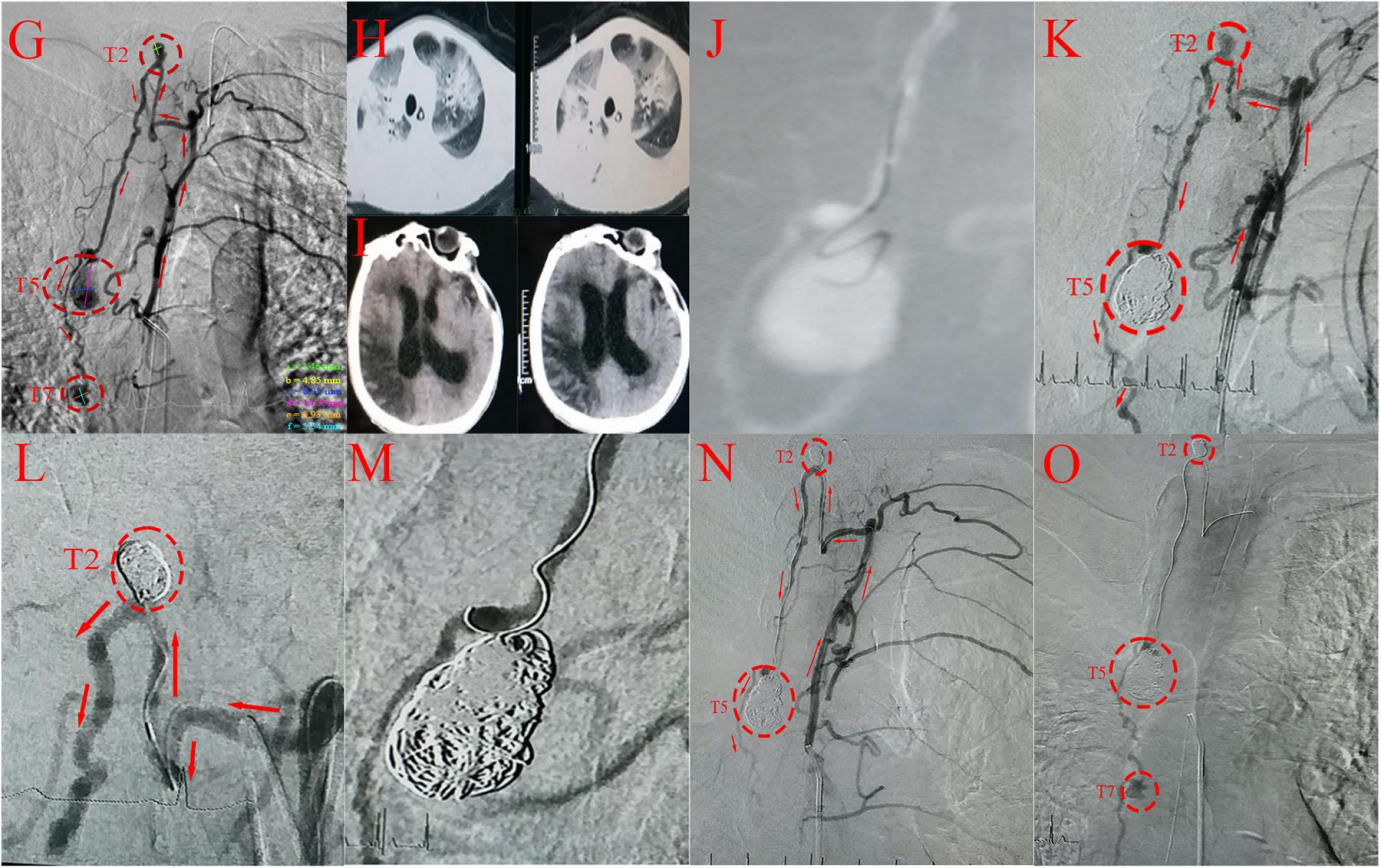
(G) spinal angiography confirming multiple tandem aneurysm-like structures in the spinal artery at the T2, T5 and T7 vertebral levels, with sizes of 3.5*4.9(saccular), 8.3*14.6(pseudoaneurysm) and 6.9*5.5(saccular) mm (red circles), and feeding artery (red arrows). (H) Lung CT revealing bilateral pneumonia. (I) Head CT showing a large area of infarction in the right cerebral hemisphere. (J) Spinal cord angiography confirming a pseudoaneurysm at the T5 level. (K,L) Embolization of T2- and T5-level aneurysms with coils (red circles). Red arrows represent the feeding artery. (M) Multiple microguidewire attempts during T7-level aneurysm embolization failing to achieve stable positioning. (N,O) Spinal cord angiography demonstrating that the aneurysms at the T2- and T5-level aneurysms were completely embolized (red circles) Red arrows represent the feeding artery. Delayed contrast opacification of the T7-level aneurysm during the late arterial phase suggesting a low rupture propensity.
